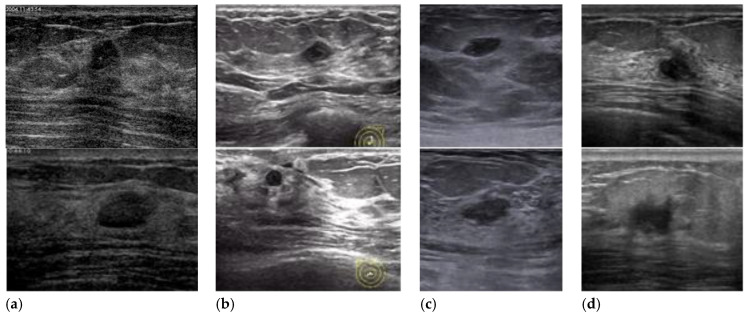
Breast ultrasound images collected using different devices. BUS images produced using (a) GE VIVID 7, (b) GELOGIQ E9, (c) Simens ACUSON S2000, and (d) Hitachi EUB-6500.
